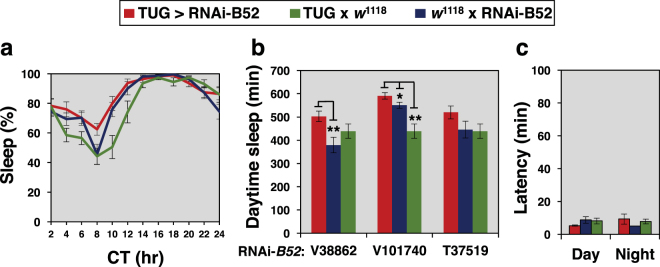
The effect of B52 on daily sleep levels is strongly reduced in constant dark conditions. (a–c) Young male progeny from the indicated cross (top of panels) were kept at 18 °C and entrained for 5 days in 12 hr:12 hr light/dark (LD) cycles followed by several days in constant darkness [where circadian time (CT) 0 is the beginning of the subjective day; i.e., herein defined as equivalent to ZT0 in LD]. Figures are derived from the same data shown in Fig. 3, except that data are from the first day of constant darkness based on averaging values obtained from 16 individual flies for each genotype. The following *p* values were determined: [Panel (a), ANOVA, TUG > V101740 compared to both controls, 0.13]. [Panel (b), two-tailed *t*-test; TUG > V38862 vs V38862 x *w*^1118^, 4.3 × 10^−3^; TUG > V38862 vs TUG x *w*^1118^, 0.11; TUG > V101740 vs V101740 x *w*^1118^, 0.022; TUG > V101740 vs TUG x *w*^1118^, 9.38 × 10^−5^; TUG > T37519 vs T37519 x *w*^1118^, 0.13; TUG > T37519 vs TUG x *w*^1118^, 0.079]. [Panel (c), two-tailed *t*-test; for day values, TUG > V101740 vs V101740 x *w*^1118^, 0.12; TUG > V101740 vs TUG x *w*^1118^, 0.16; for night values, TUG > V101740 vs V101740 x *w*^1118^, 0.15; TUG > V101740 vs TUG x *w*^1118^, 0.65]. The corresponding daily activity profile for panel (a) is shown in figure S2b.
